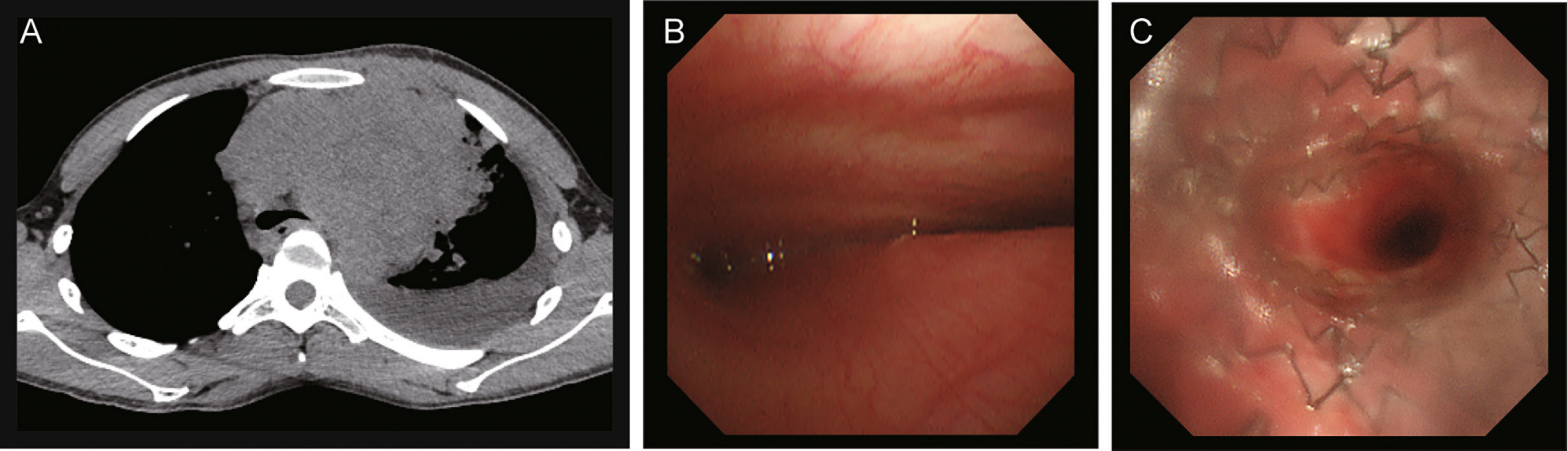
Findings before and after stent placement. (A) Non‐contrast chest computed tomography (CT) at presentation showing an anterior mediastinal mass causing external compression and complete obstruction of the left main bronchus. (B) Bronchoscopic view at the carina level revealing significant luminal narrowing from extrinsic compression, with both main bronchi obscured. (C) Bronchoscopic image demonstrating successful hybrid stent deployment with restored patency of the right main bronchus.
